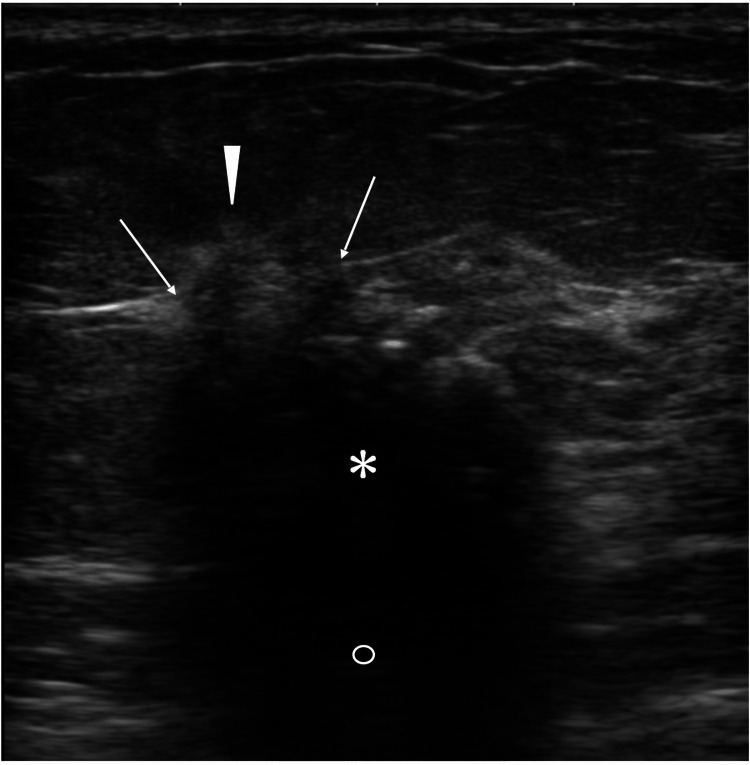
Ultrasound findings Ultrasound showed an oval mass (asterisk) with indistinct margins, internal low echoes, attenuated posterior echoes (open circle), disruption of mammary gland anterior borders (arrows), and focal haloes (arrowhead)
